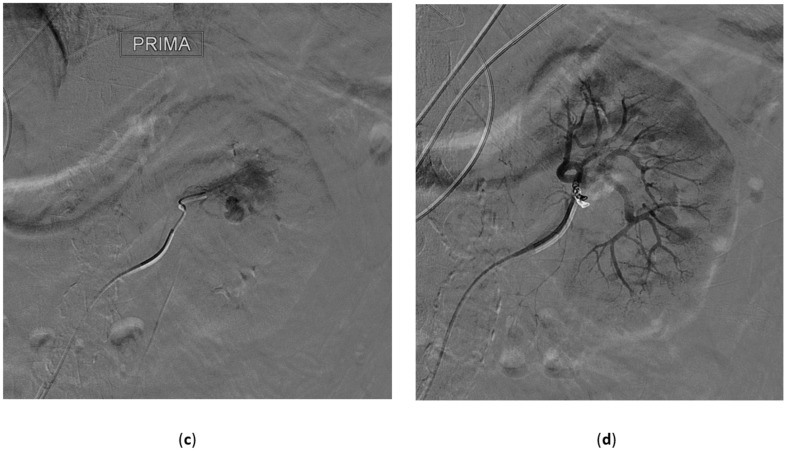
Renal trauma 59-year-old male. Car accident, hematuria. (a,b) Contrast-enhanced CT shows a left perirenal hematoma with an active bleeding inside; (c) Superselective angiography confirms the bleeding, which was treated by deployment of a microcoil (d).
